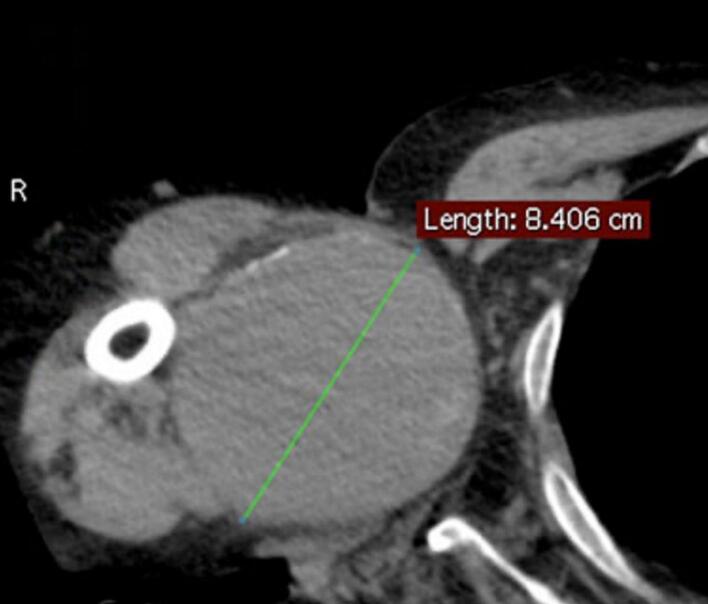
Basal CT scan showing huge brachial artery aneurysm with maximum diameter of 8.4 cm.
